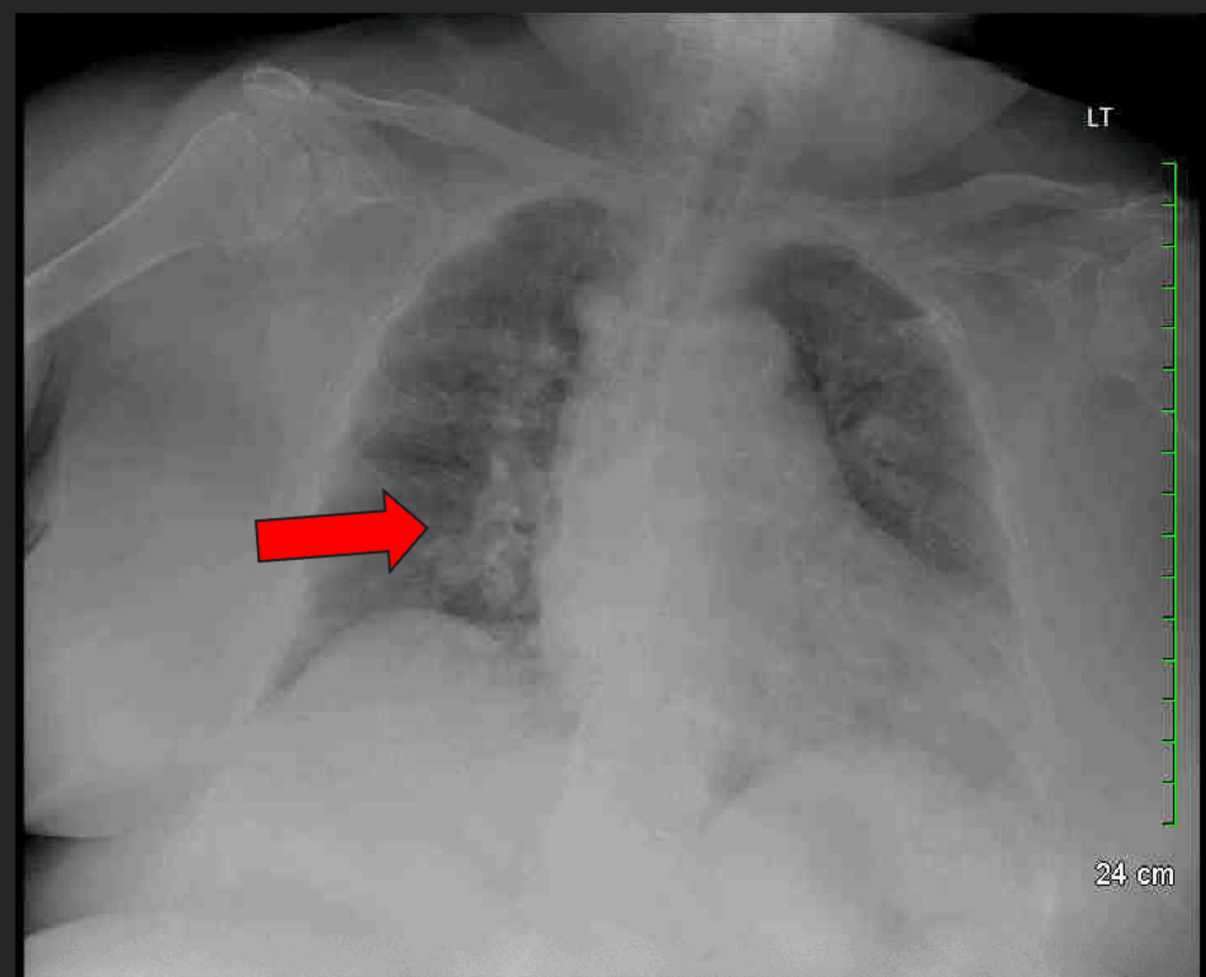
Chest X-ray from two weeks prior. The arrow indicates the location and appearance of the opacities with no significant change, suggesting a chronic or recurrent process.
